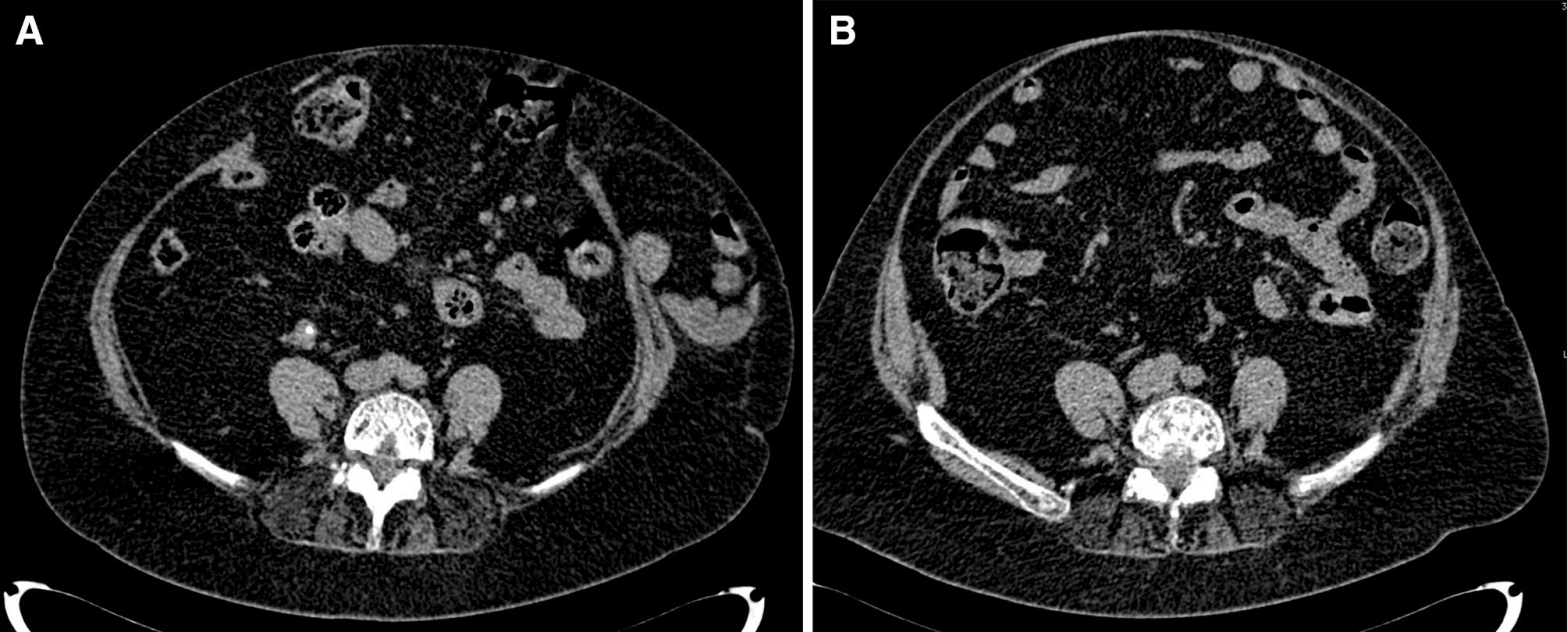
a Preoperative CT scan of an old lady with a midline defect 30 cm long and maximum 20 cm width. b Control CT scan in Valsalva at 18 postoperative months of the patient a after AWR with the combination of TAR and external oblique release. A weak but continent abdominal wall is observed
